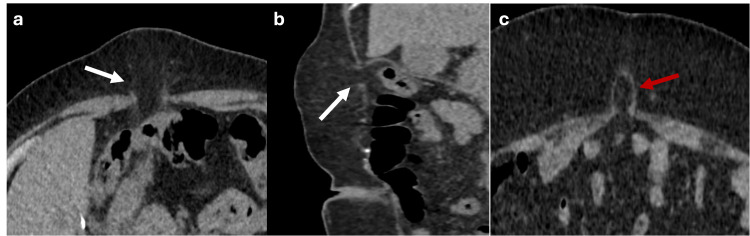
Axial and sagittal non-contrast abdominal CT scan showing an epigastric and umbilical hernia (a, b) Axial and sagittal CT showing a fat-content epigastric hernia located between the xiphoid process and the umbilicus (white arrows). (c) Axial non-contrast abdominal CT scan showing a hernia sac with fat content protruding from the umbilical ring (red arrow), consistent with an umbilical hernia. The figure was created by the authors.
